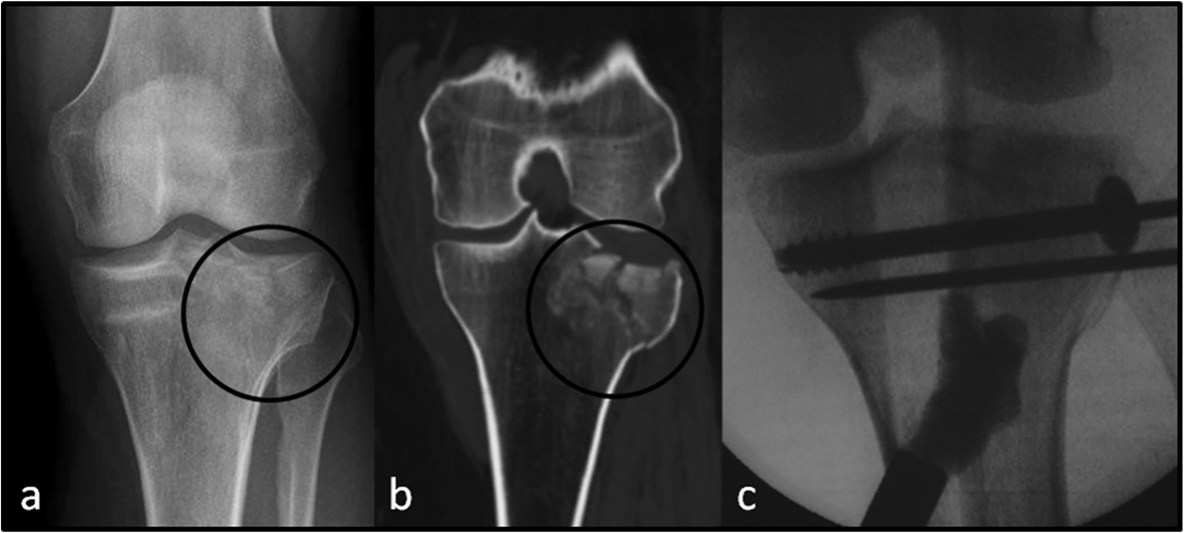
A 35 year old female with a Schatzker II, Müller AO grade 41-B3 fracture. (a) Pre-operative X-rays; (b) Pre-operative CT-scan; (c) Intra-operatively CERAMENT™ was injected under fluoroscopy following closed reduction and before fixation with two cannulated cancellous bone screws
